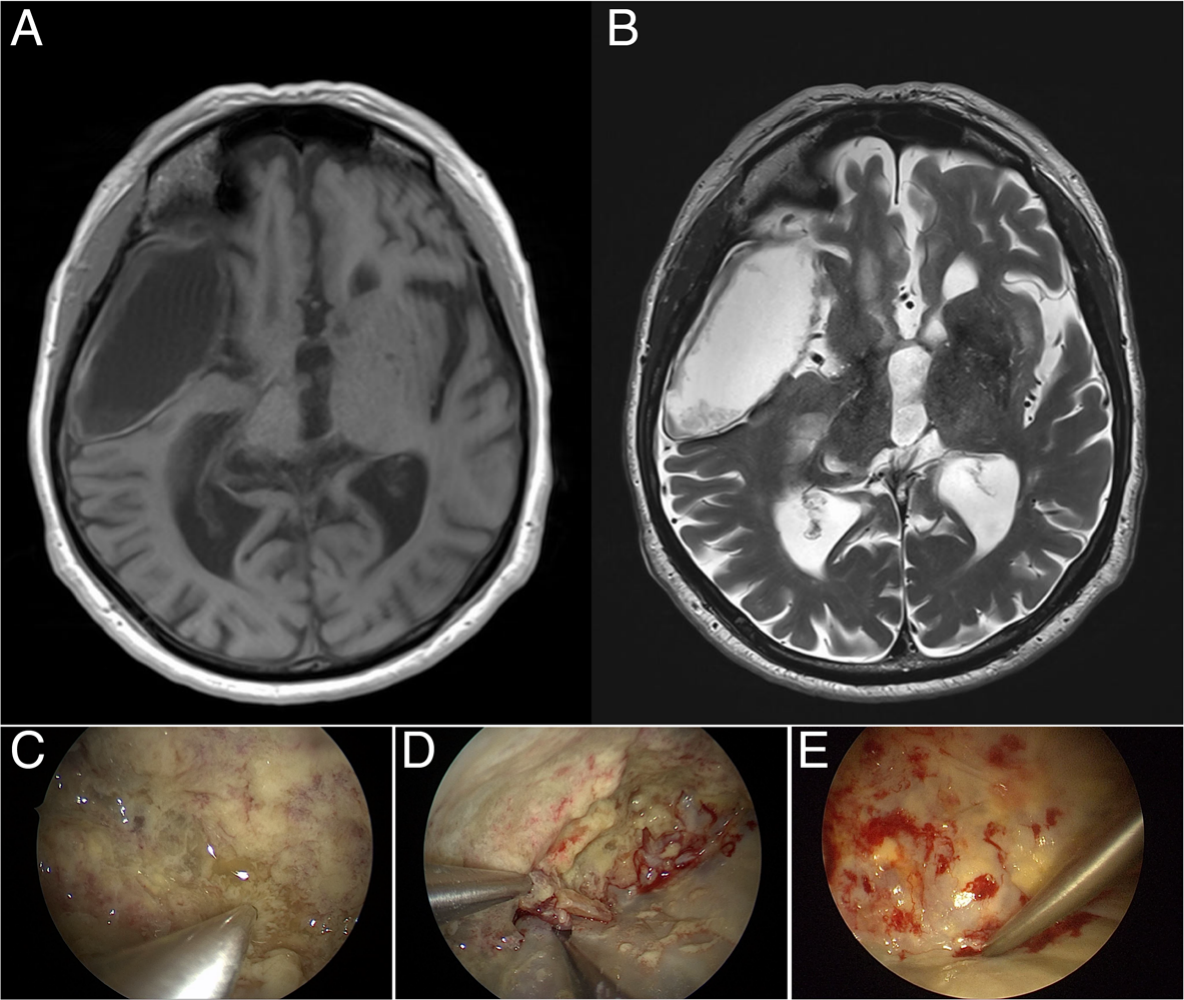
T2-weighted MRI with right temporal arachnoid cyst with signs of intracystic hemorrhage (a). Postoperative control-MRI shows total resection of the tumoral tissue within the cyst (b). Initial intraoperative endoscopy showed a thick, yellowish lining of the inner wall of the cyst, (c) which was removed under endoscopy (d) with eventual total resection (e)
